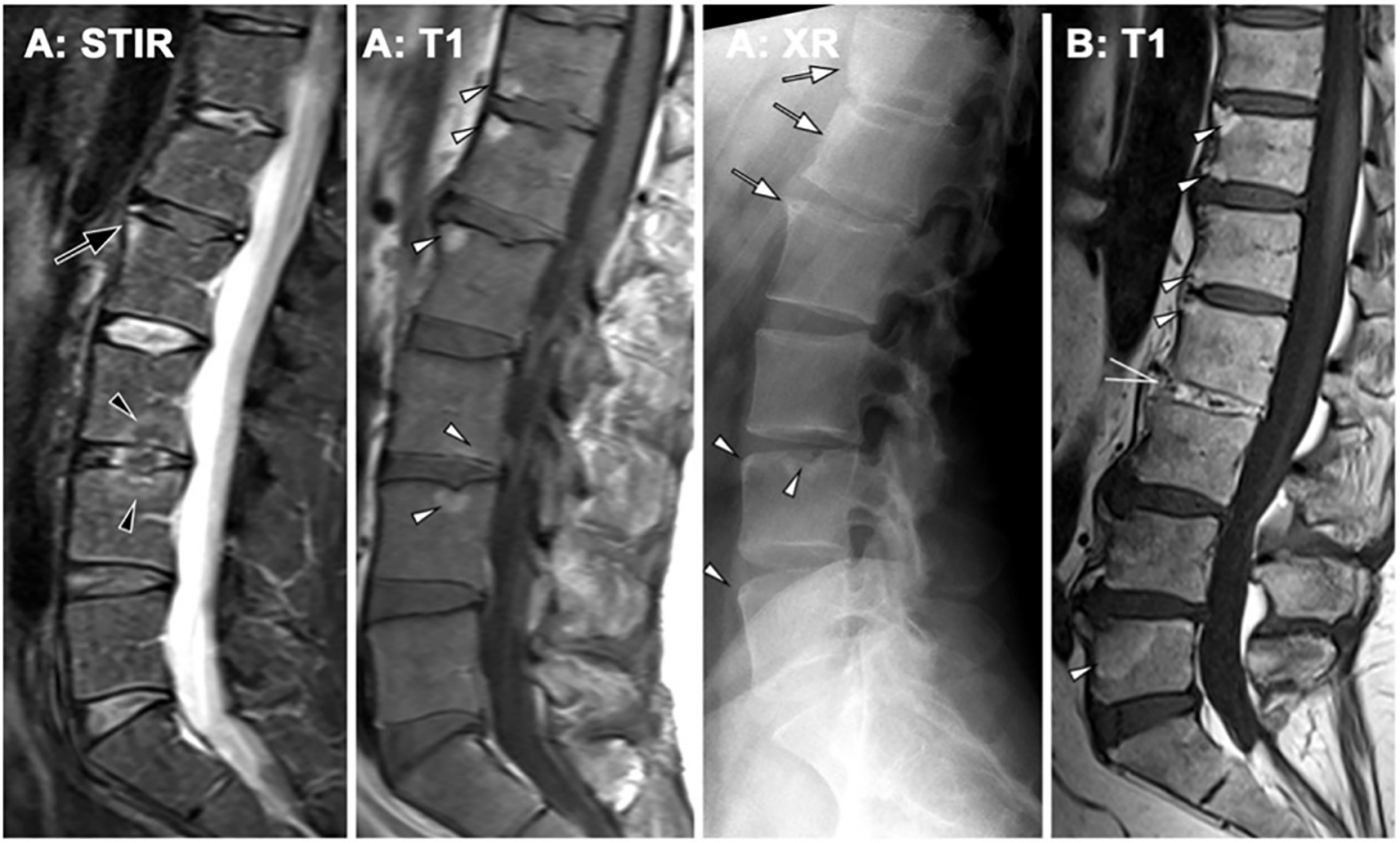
Axial spondyloarthritis (axSpA) at the spine. (A) A 42-year-old male patient showing inflammatory corner lesions (black arrow) and endplate lesions (black arrowhead) in STIR and corresponding fat lesions in T1. Radiography (XR) shows new bone formation with box-shaped vertebra and shiny corners (white arrows) and erosions (white arrowheads). (B) A different patient with advanced axSpA shows fat lesions (white arrowheads) and transdiscal ankylosis (open white arrow).
